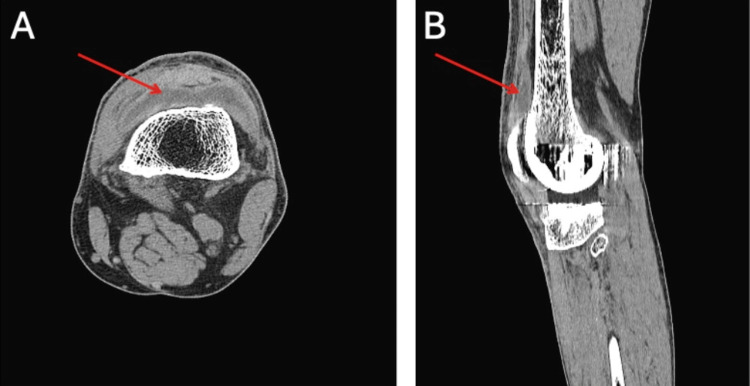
CT scan of the left knee. A: Axial view. B: Sagittal view. CT scan of the left knee demonstrating a large complex effusion with density higher than simple fluid (arrows), concerning for infection versus hemorrhage.
